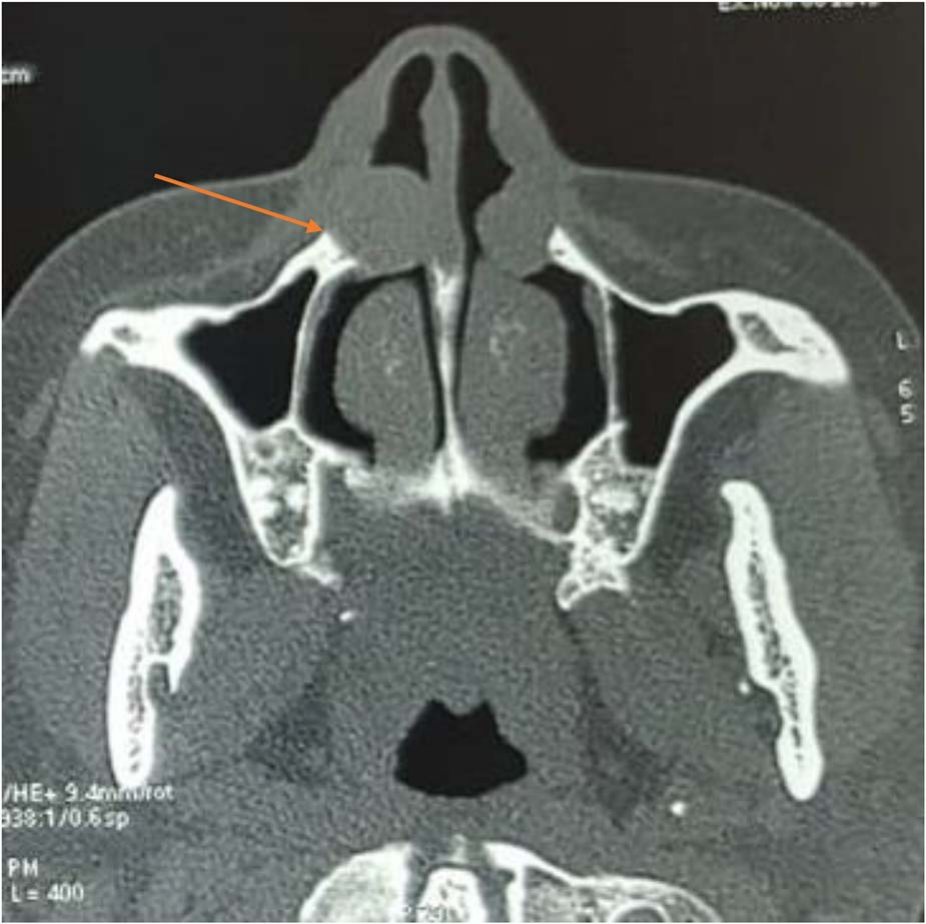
Axial computed tomography showing a bilateral well-defined, hypodense, extraosseous lesion near the ala nasi; note the cortical scalloping on the right side (arrow).
